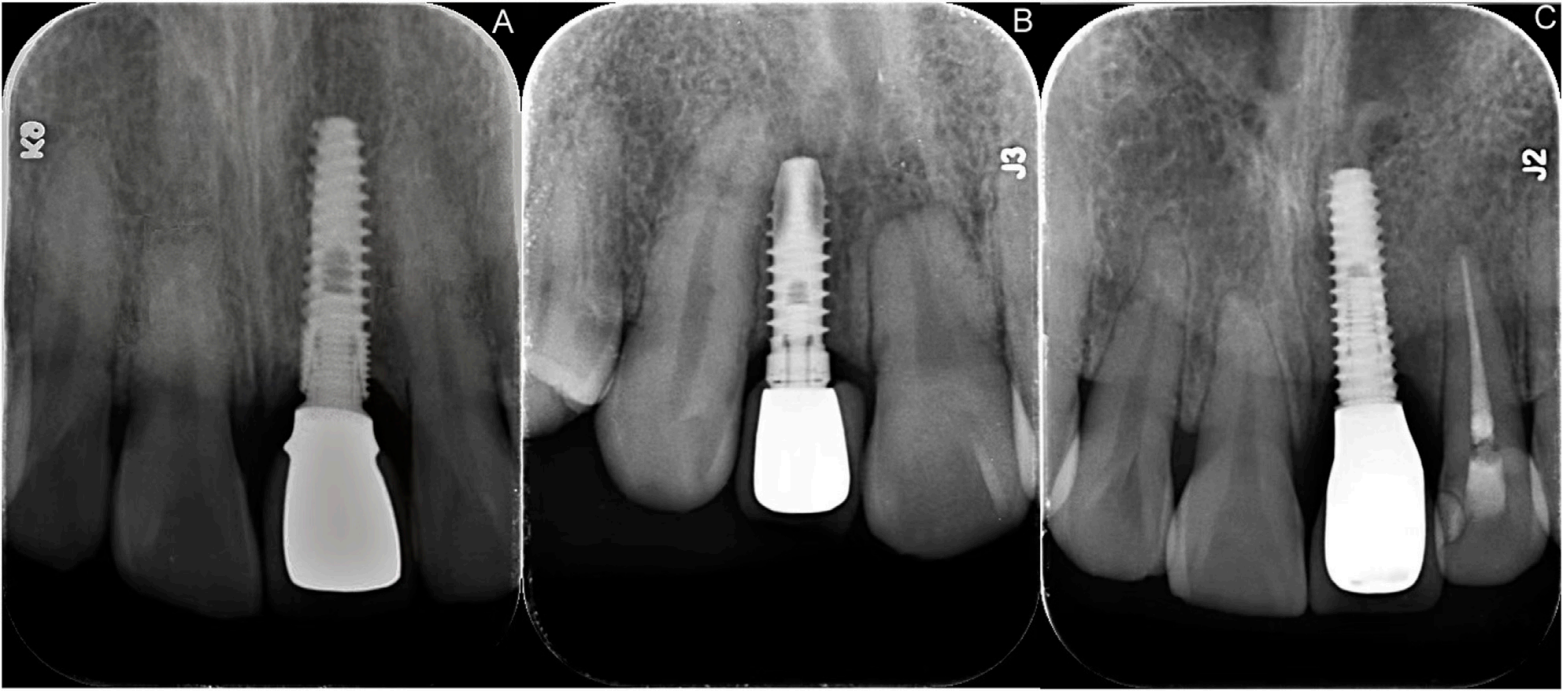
Representative periapical radiographs showing MBL at the 24-month follow-up for the three implant systems. (A) Straumann^®^ BLT implant. (B) Thommen SPI^®^ ELEMENT implant. (C) Osstem TSIII SA implant.
